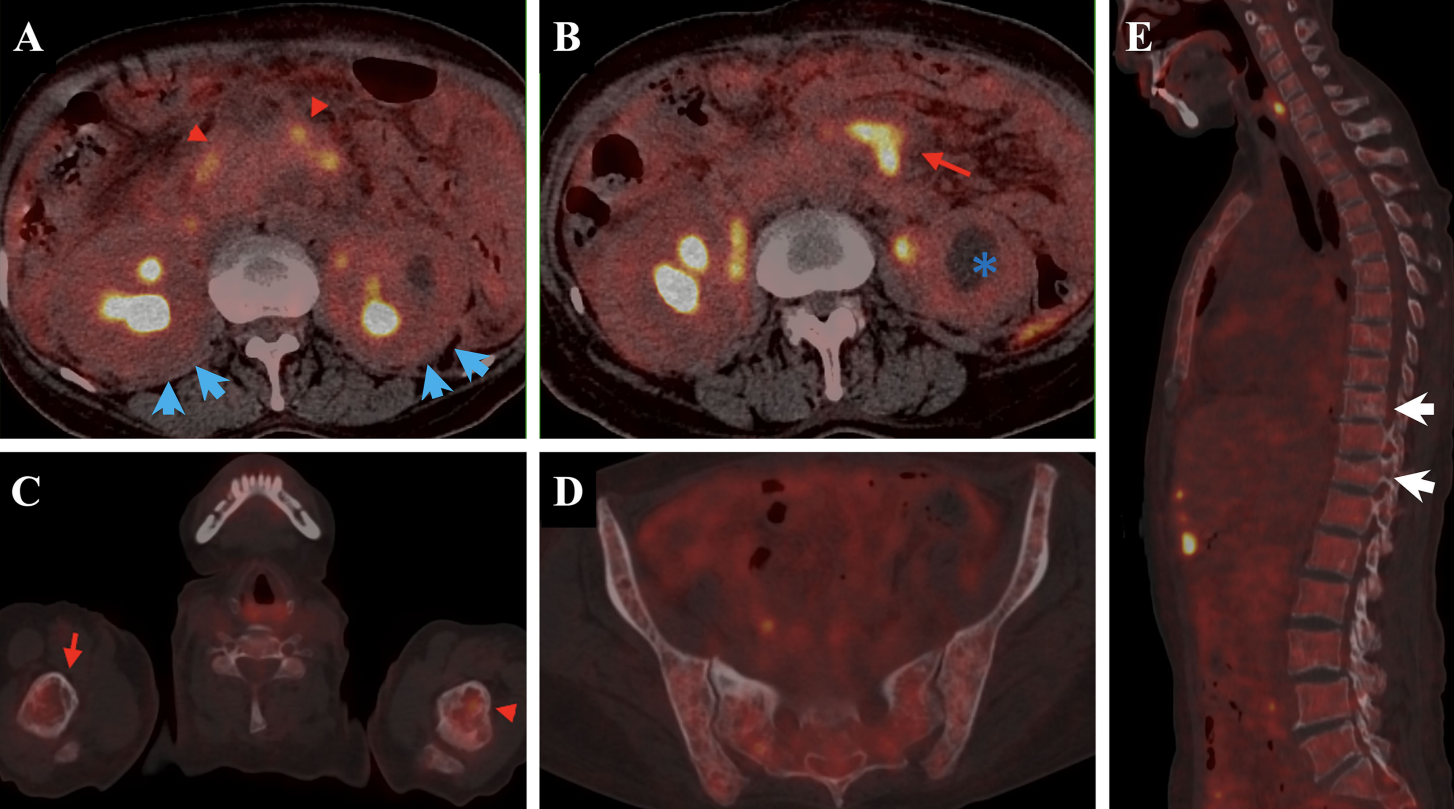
The patient’s PET-CT demonstrates involvement of multiple tissues and organs. Notably, pancreatic abnormalities [(A), red triangle] and bilateral renal enlargement with hydronephrosis [(B), blue asterisk] are observed, along with mild “hairy kidney” appearance [(A), blue arrow]. There are soft tissue density shadows adjacent to the abdominal aorta in the retroperitoneum and bilateral iliac vessels [(B), red arrow], all exhibiting increased glucose uptake. Additionally, uneven bone density is observed in the spine and pelvic bones, with multiple patchy low-density areas (C–E). Compression fractures are noted at the T9 and T11 vertebral.
